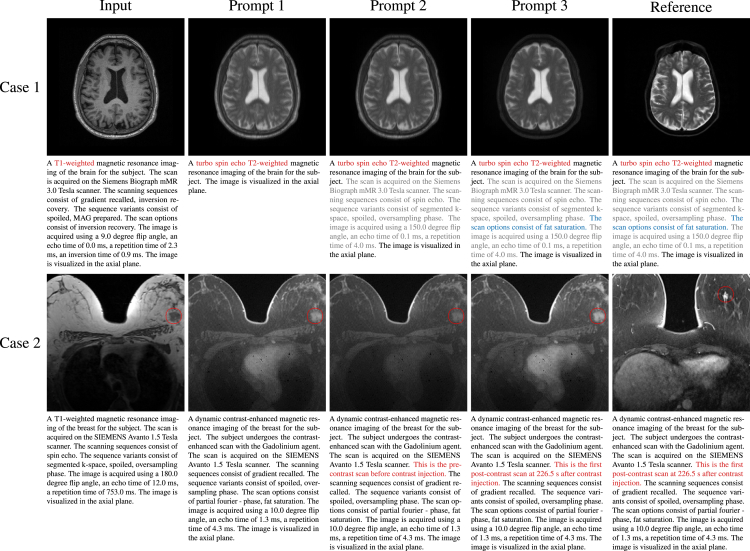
Axial visualization of the impact of changing prompts on generated images In case 1, altering the sequence (prompt 1) results in a noticeable change in the appearance of the image. Including additional scan information in prompt 2 has a minimal impact on the appearance. Fat saturation (prompt 3) further suppresses the intensity of fat tissue under the skin, thus rendering the generated image more consistent with the reference image. In case 2, when the specific time point of DCE-MRI is not specified (prompt 1), the intensity enhancement of the cardiac and tumor (red circle) is between the time points before contrast (prompt 2) and the first post-contrast (prompt 3). Note that the proposed model requires only the input image and the target prompt. The reference image is not used as an input but is provided solely for comparison. It corresponds to the given prompt but is from a different subject because the input case lacks the corresponding image of the reference prompt.
